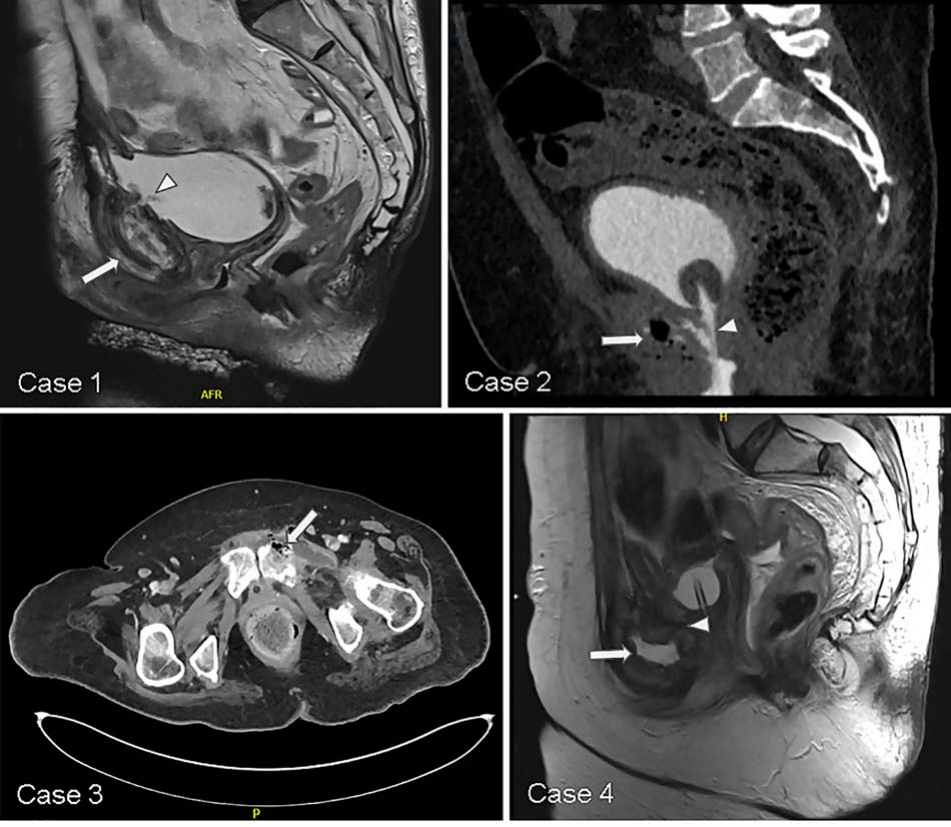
Representative CT and MRI imaging demonstrating USF (white arrowhead) and features suggesting pubic osteomyelitis (white arrow) in each respective case.
